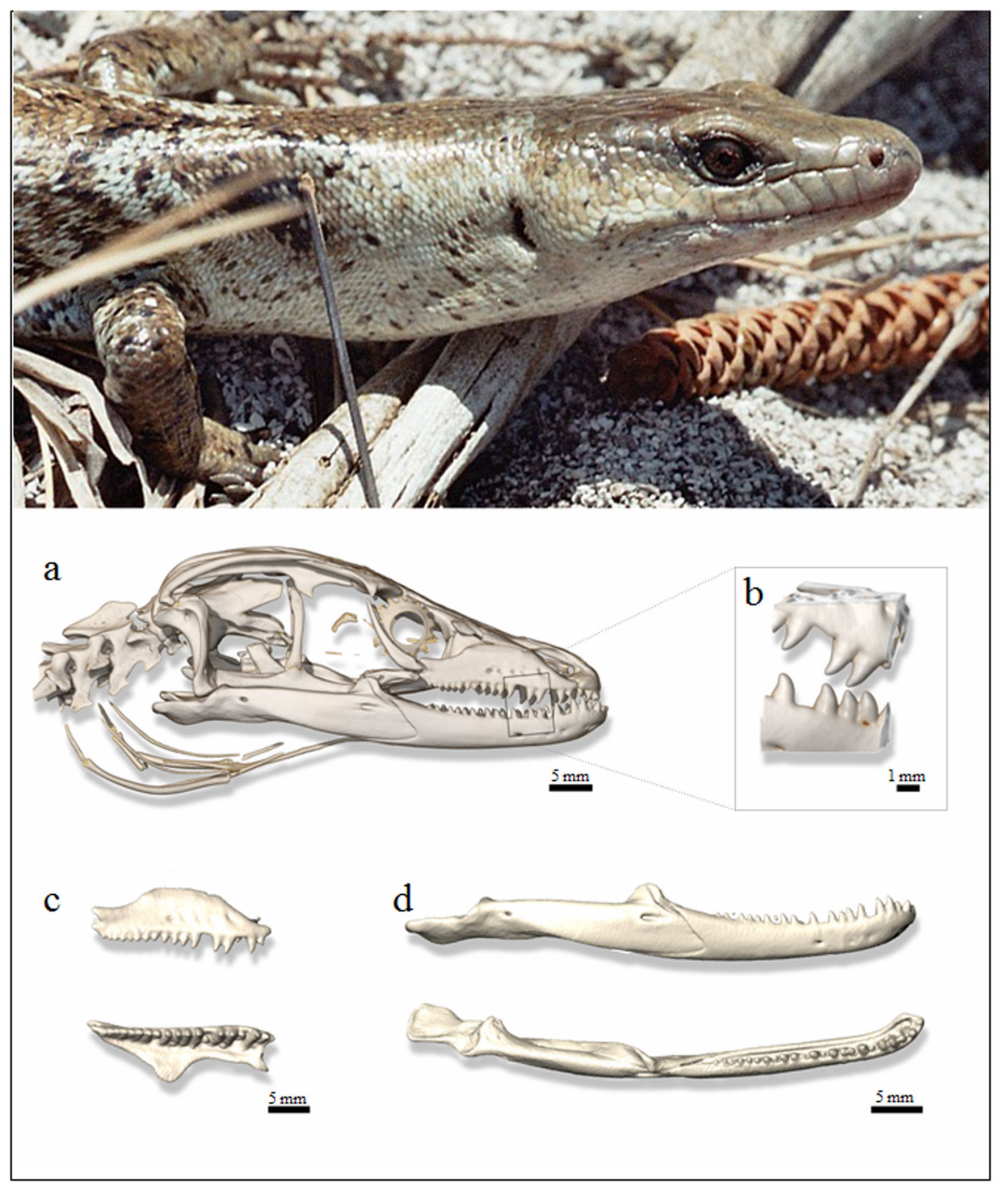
X-ray microtomography and 3D visualization. Top: anterior view of the body of the *Phoboscincus bocourti* captured during the November 2005 expedition to Brosse Islet. Bottom: volume rendering of the skull of the *P. bocourti* holotype - (a) Lateral view of skull including first vertebrae and hyoid apparatus; (b) Magnification of dentary and maxillary areas; (c) Morphology of dentary and maxillary teeth; (d) Lateral and dorsal view of dentary.
